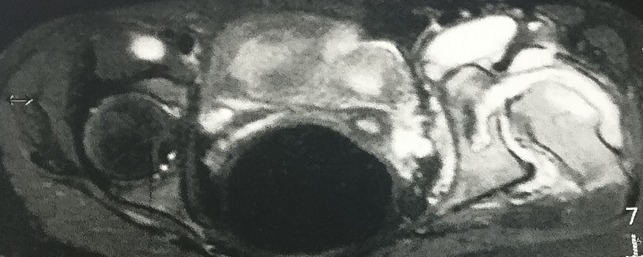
Radiograph demonstrating the axial T2 fat saturation anterior fluid collection.
